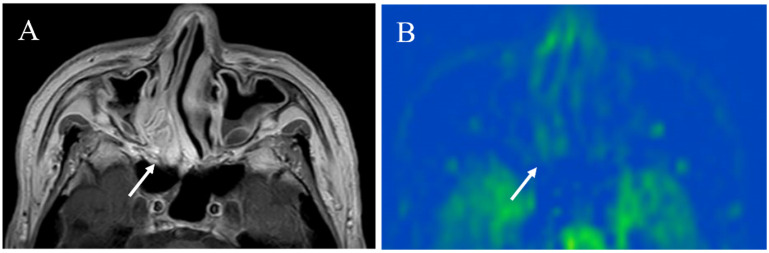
A 73-year-old male with inverted papilloma: (A) Contrast-enhanced T1-weighted image shows an enhanced mass with convoluted cerebriform pattern (arrow); (B) pCASL image shows a slight increase in blood flow within the lesion (arrow) (TBF mean of 39.46 mL/100 g/min).
